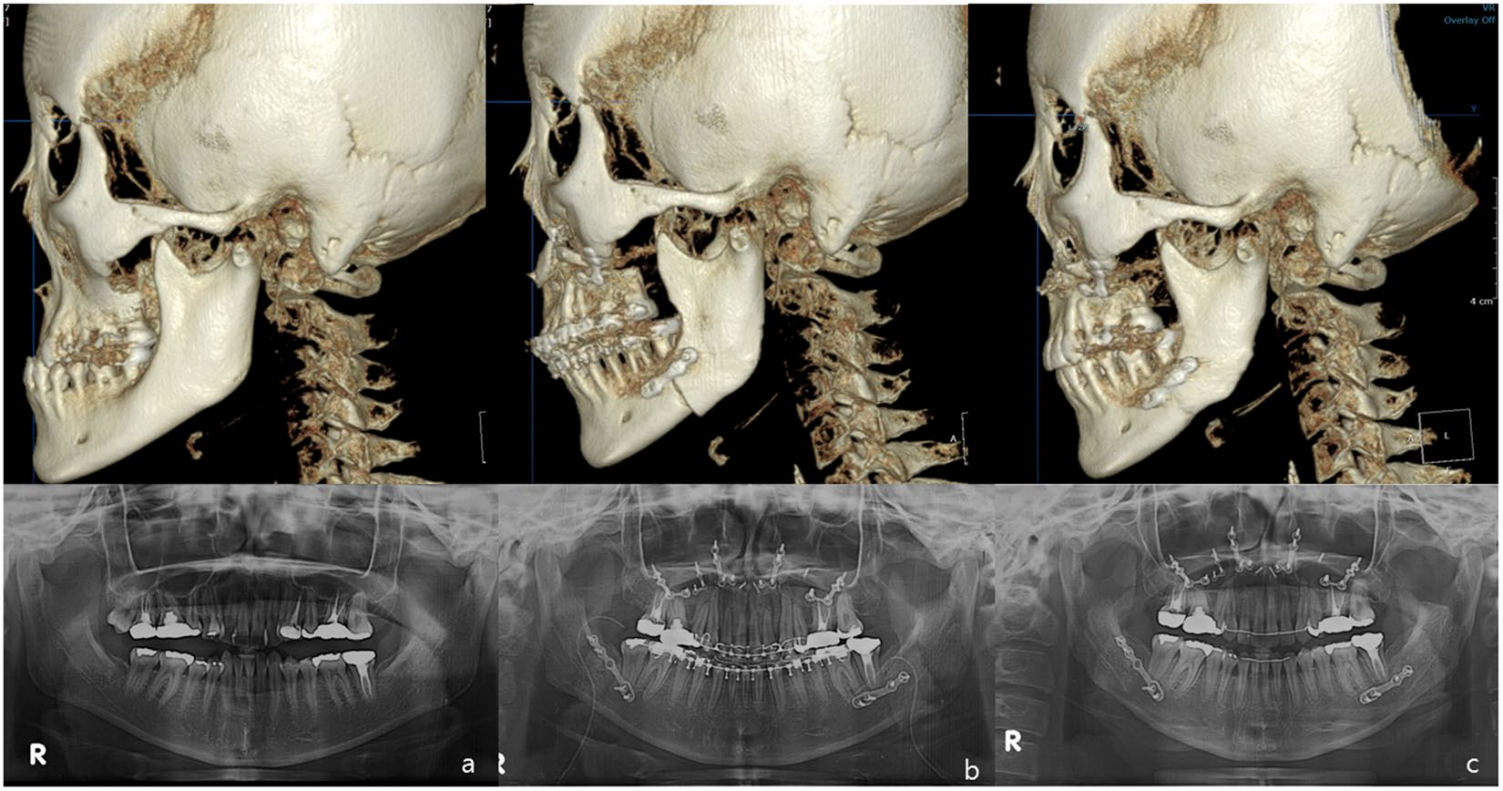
Three-dimensional ConeBeam CT and Panoramic radiographs demonstrating the use of a YK- plate after SSO. (a) Preoperative view, a 23-year-old patient with mandibular prognathism (b) 2 days after the operation, Bimaxillary operation was done. YK-plates were used on mandible (c) four months after the surgery, the bone remodeling is in progress, and the YK-plates are stable.
